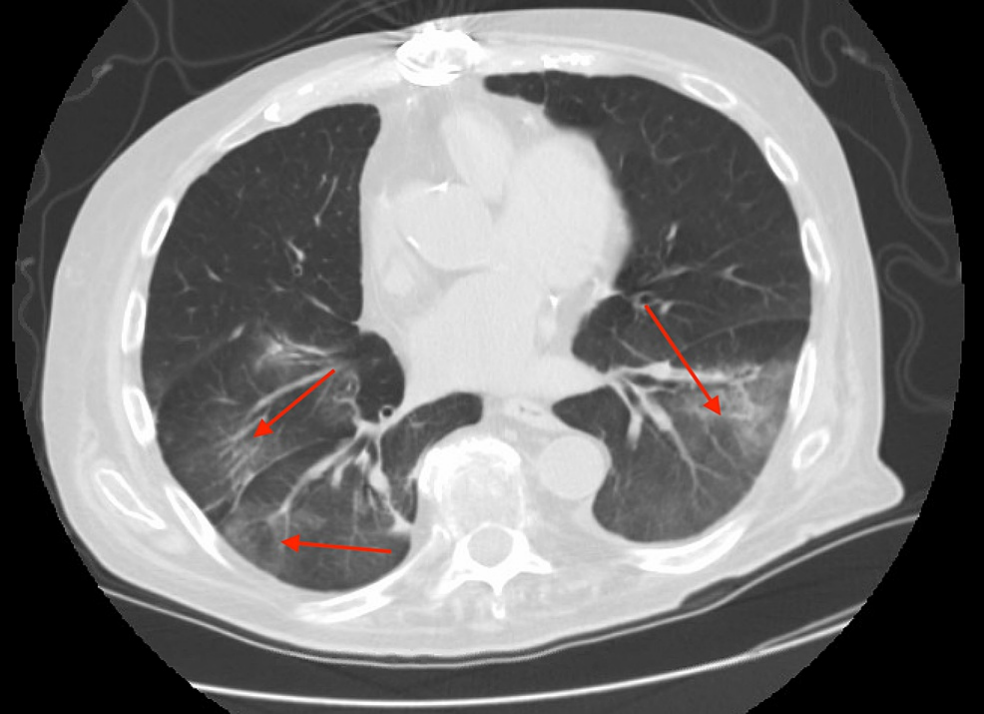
Chest CT showing patchy bilateral ground-glass infiltrates (arrows)
